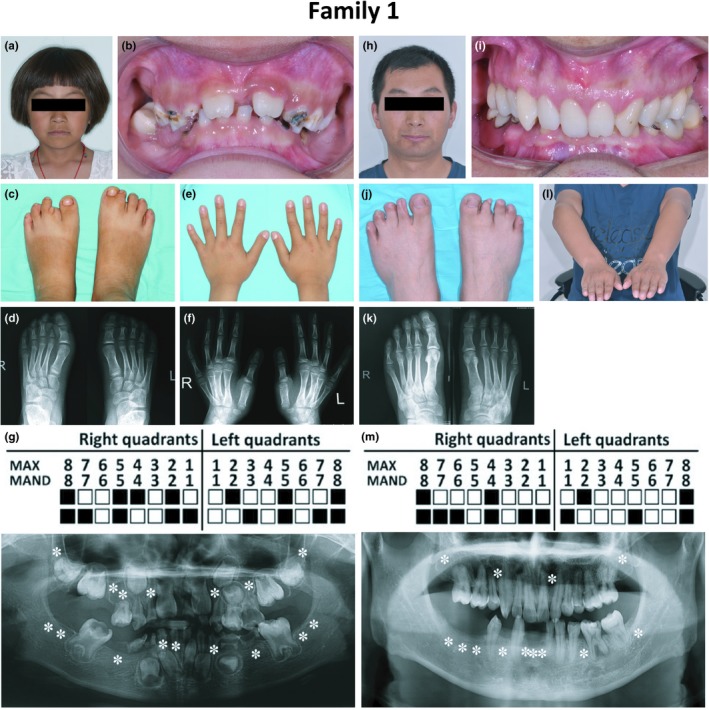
Representative clinical observations from photographic and x‐ray data of Family 1. Proband #1 (a) presented ectodermal phenotype with missing teeth (b) and nail dysplasia (c). Examination revealed cutaneous syndactyly between the first and second toes of the right foot and a missing distal phalanx of the second toe of the left foot (c,d). She had normal hands (e,f). Panoramic radiography showed tooth agenesis and taurodontism of the mandibular first molar (g). Her father (h) presented with tooth agenesis (i, m) and nail dysplasia (j). We found cutaneous syndactyly between the third and fourth toes of both feet and an abnormal second toe on the right foot (j,k). Physical examination revealed cubitus valgus deformity in the right arm (l)
